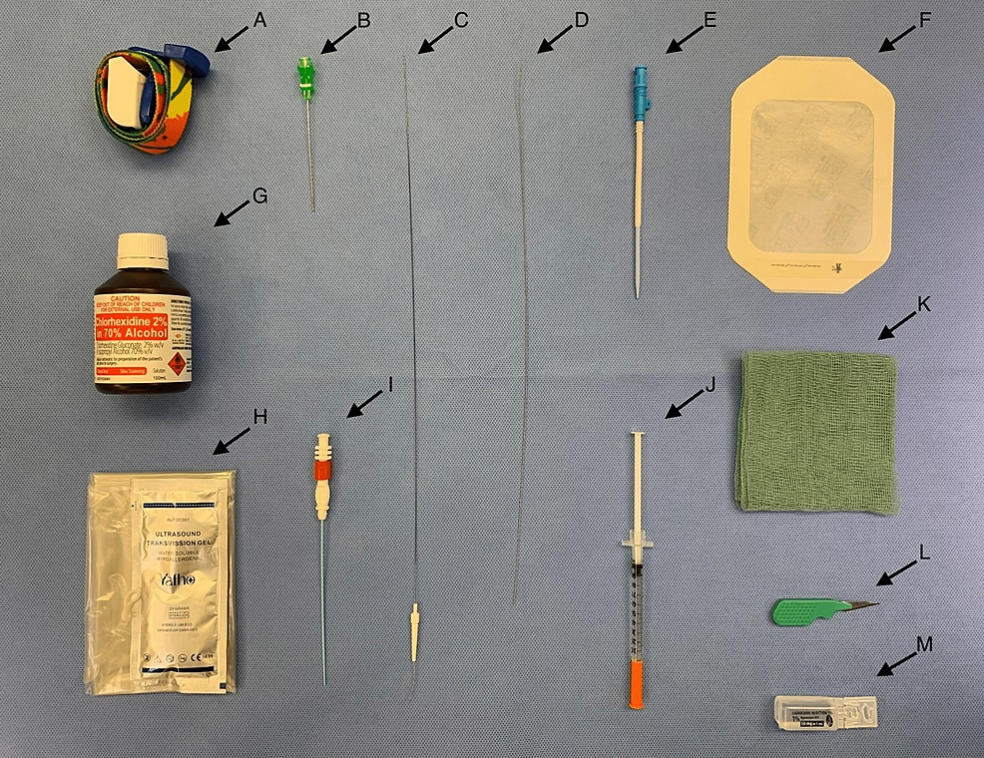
Equipment required for ultrasound-guided insertion of a micropuncture introducer catheter and conversion to a rapid infusion catheter (RIC). (A) Tourniquet. (B) 21-Ga 7 cm introducer needle with echogenic tip. (C) 0.018-inch diameter, 40 cm nitinol guidewire with floppy radiopaque tip. (D) Rapid infusion catheter (RIC®) 0.025-inch diameter, 13-1/8-inch (33 cm) spring-wire guide.  (E) 8.5-Fr 2-½-inch (6.4 cm) Arrow-Flex® radiopaque RIC® catheter with tissue dilator. (F) Large transparent dressing. (G) Chlorhexidine 2% in 70% alcohol antiseptic skin preparation solution. (H) Ultrasound probe cover. (I) 4-Fr micropuncture introducer catheter with a coaxial dilator. (J) Insulin syringe for local anesthetic for skin infiltration. (K) Gauze. (L) #11 scalpel. (M) 1% lignocaine. (N.B. While an Argon Medical V•Stick™ Vascular Access Set is used in this image, however, a Cook® Micropuncture® Access Set can also be used).
